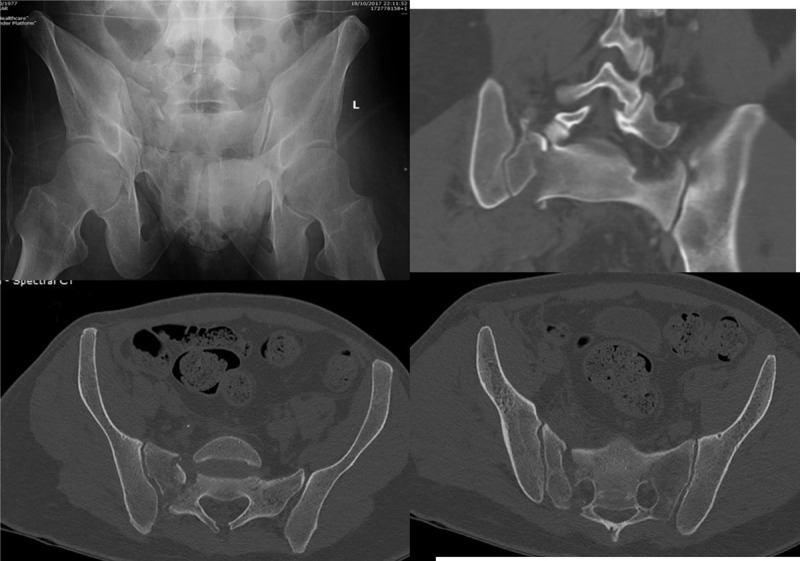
A displaced vertical shear fracture of a 40-yr-old laborer who fell from height—the outlet (top left), view, coronal, and axial CT demonstrate severe displacement. The reduced fracture is seen in Figure 2.
